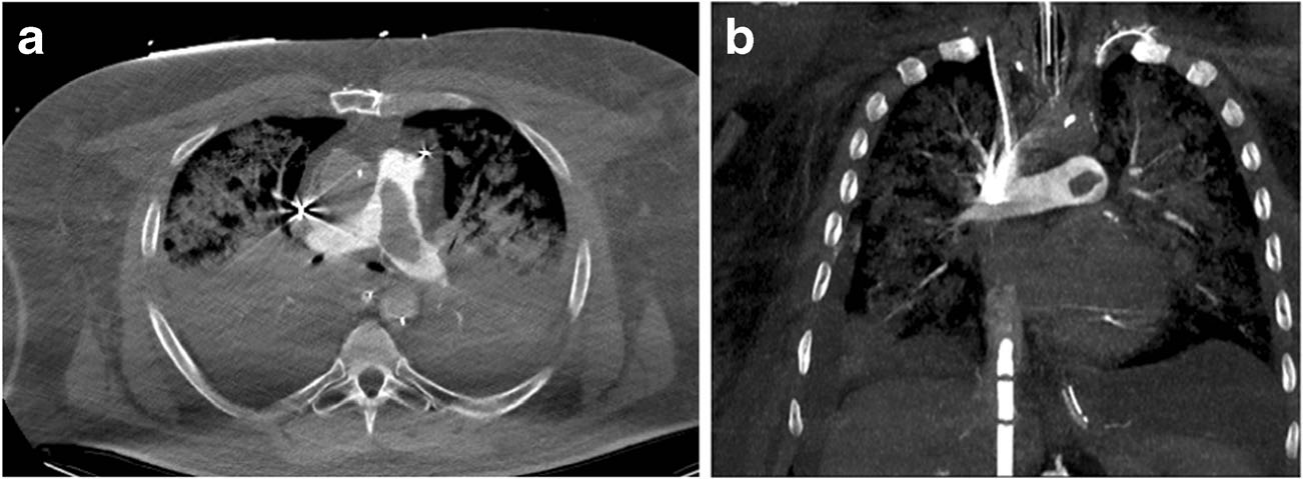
Axial CTA images from the same patient as in Fig. 17 obtained after the ECMO circuit was put on minimal flow status for a short period (25 s) to allow for near physiologic circulation. Axial (a) and coronal reformatted pulmonary CTA (b) subsequently demonstrates a large central pulmonary embolism
